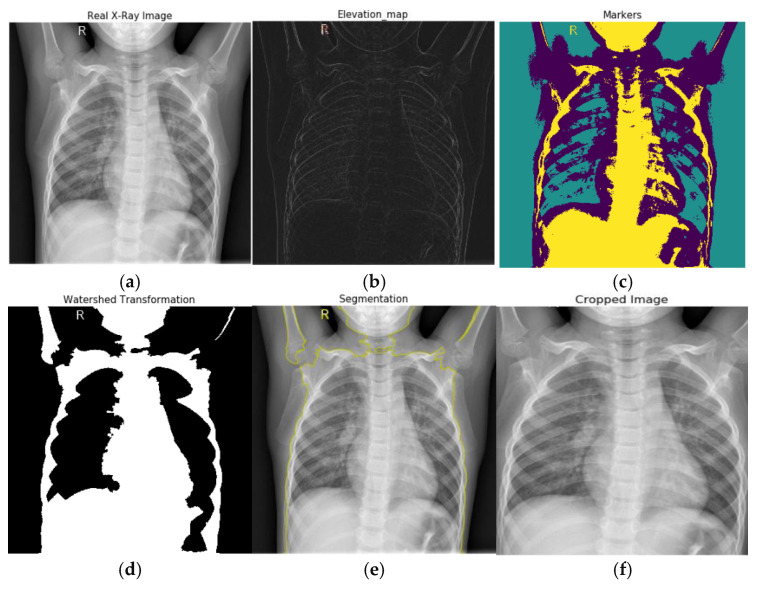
Watershed based region growing segmentation: (a) real X-ray image, (b) Sobel edge detection and elevation map, (c) setting markers, (d) watershed transformation, (e) plotting overlays, for segmentation, and (f) image cropping.
